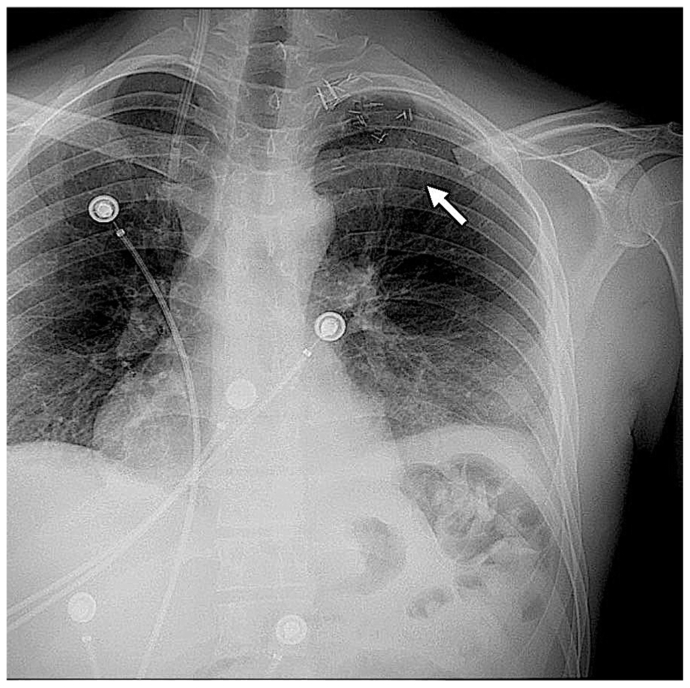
Postoperative day 3 chest radiograph demonstrating the resected clavicle and claviculectomy results (*arrow*).
